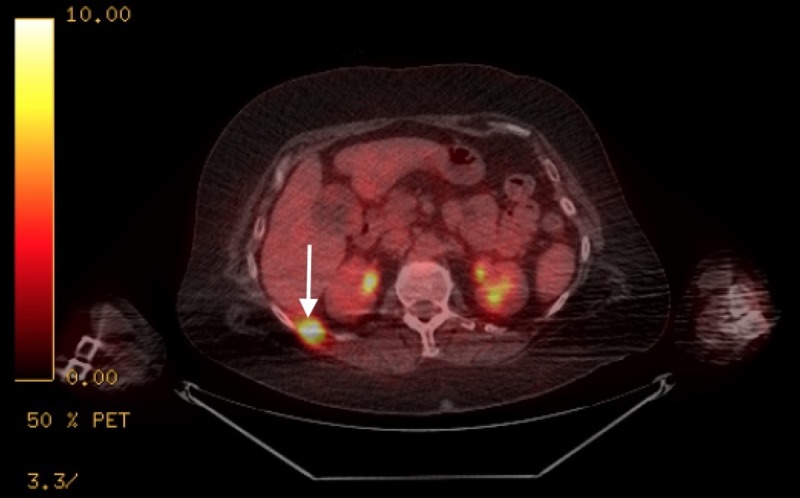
Positron emission tomography-computed tomography (PET/CT) showing area of hypermetabolic activity in the right rib.
